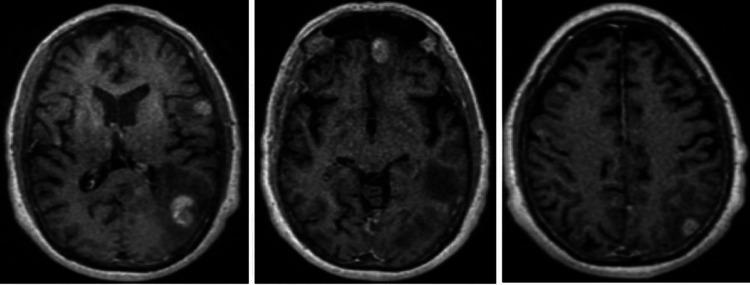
Brain MRI revealing multiple heterogeneously enhancing metastatic lesions.
